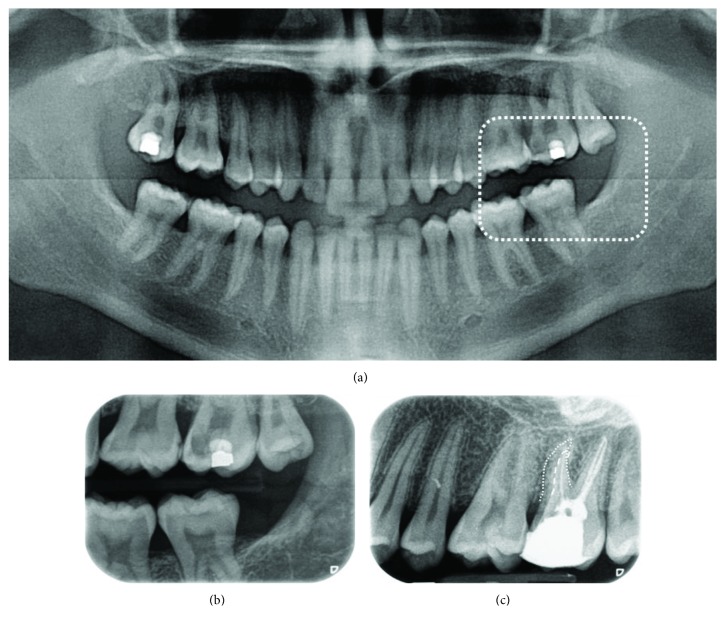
(a) Panoramic radiograph of the referred patient. (b) Bitewing view of the interesting tooth, which showed a deep carious lesion. (c) X-ray after the first endodontic treatment. In the distal roots, two root fillings are visible, whereas only one filled root canal can be identified in the mesial roots. Note two mesial roots. Buccal: dotted line; palatal: scored line.
